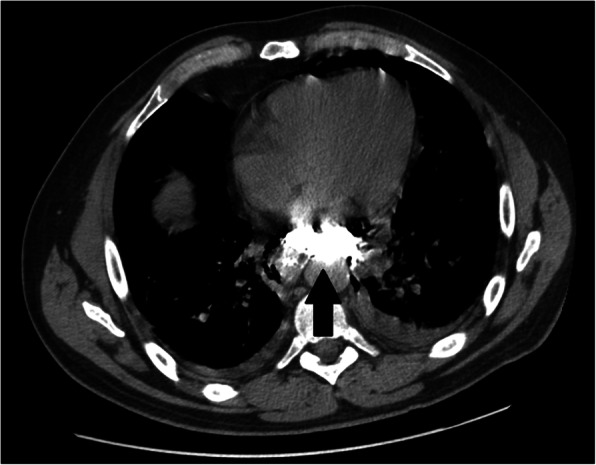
Computed tomography chest demonstrated extraluminal contrast extending into the mediastinum (arrow). Axial view
